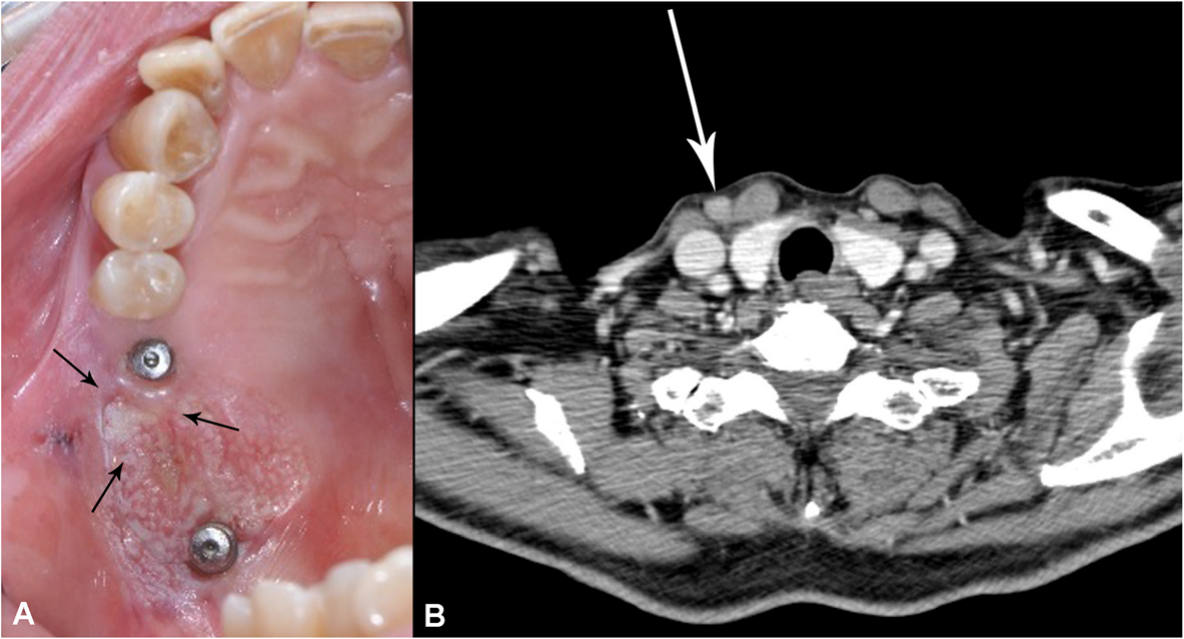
Clinical signs showed the recurrence of tumor. a Newly developed maxillary peri-implant oral malignancy lesion, b CT findings suspected of metastatic lymph node
